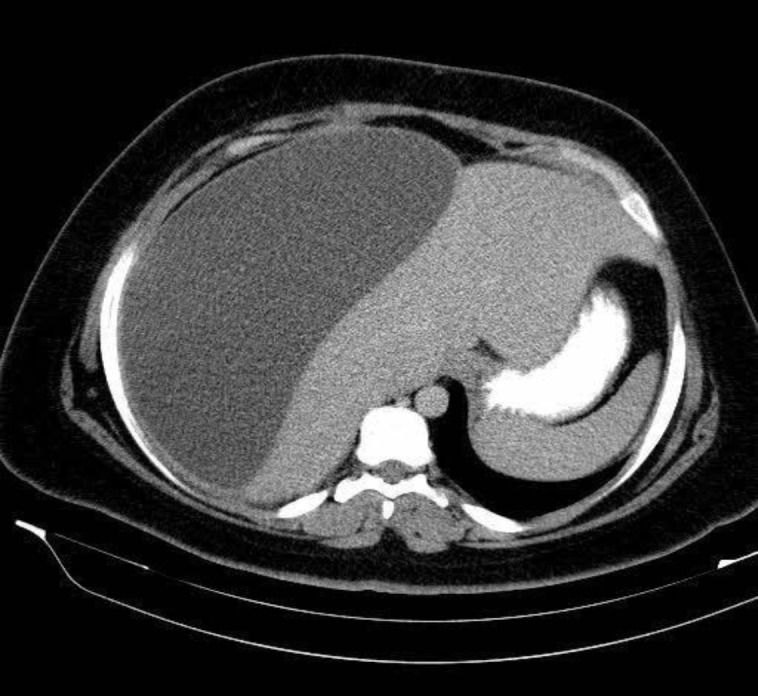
Abdominal CT (axial) demonstrated a large subcapsular biloma in the right lobe of the liver. Notable compression of underlying parenchyma is evident.
